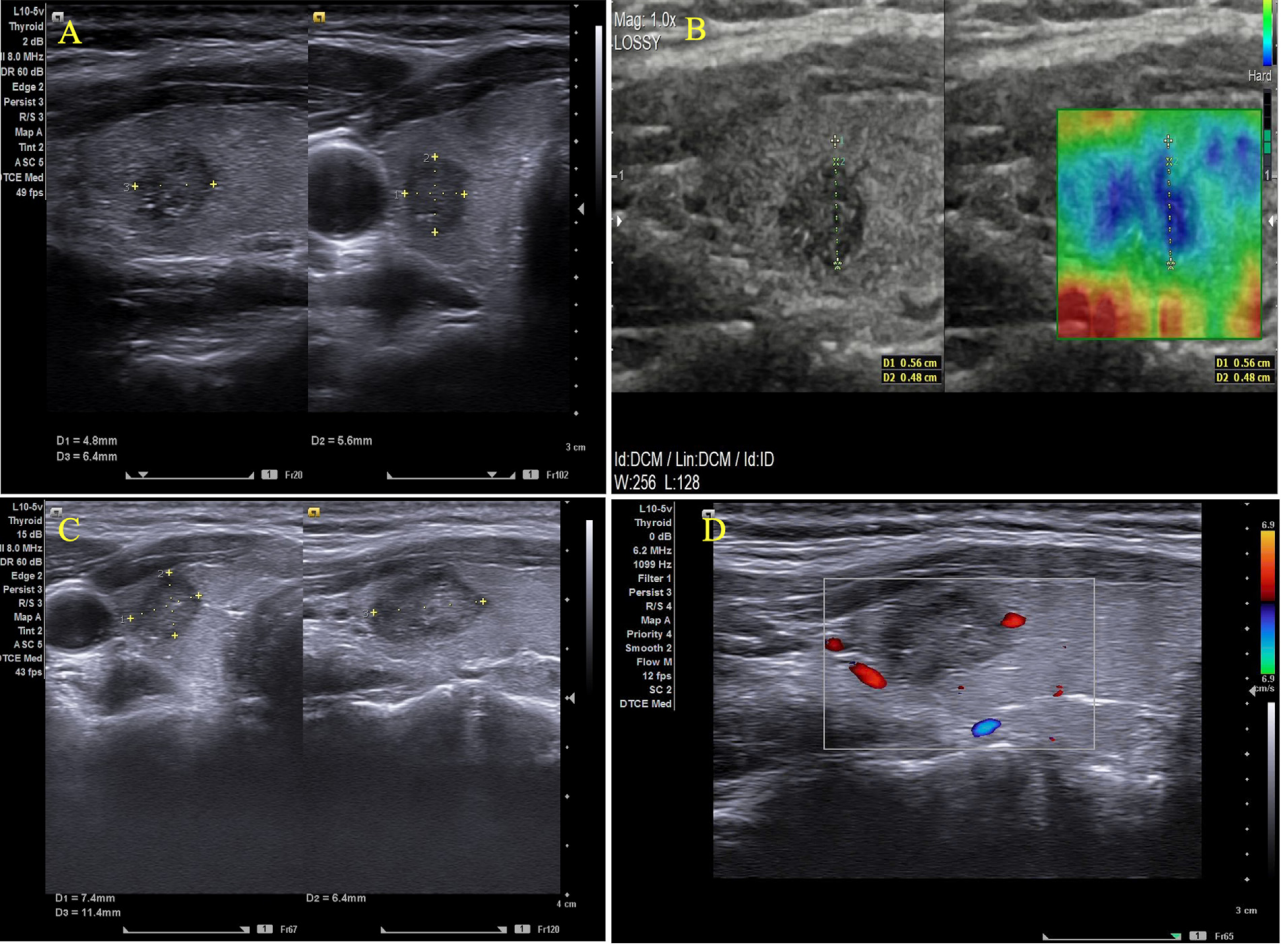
A 48-year-old woman presented to an endocrinologist following the incidental detection of a small thyroid nodule. (A) Thyroid ultrasound shows an ACR-TIRADS 5 nodule in the right lobe, measuring 4.8 × 5.6 × 6.4 mm. (B) Elastography reveals the entire lesion in hard blue with a strain ratio of 2.5. (C, D) A necrotic area measuring 7.4 × 6.4 × 11.4 mm is visible, with the surrounding thyroid tissue showing homogeneous echogenicity.
